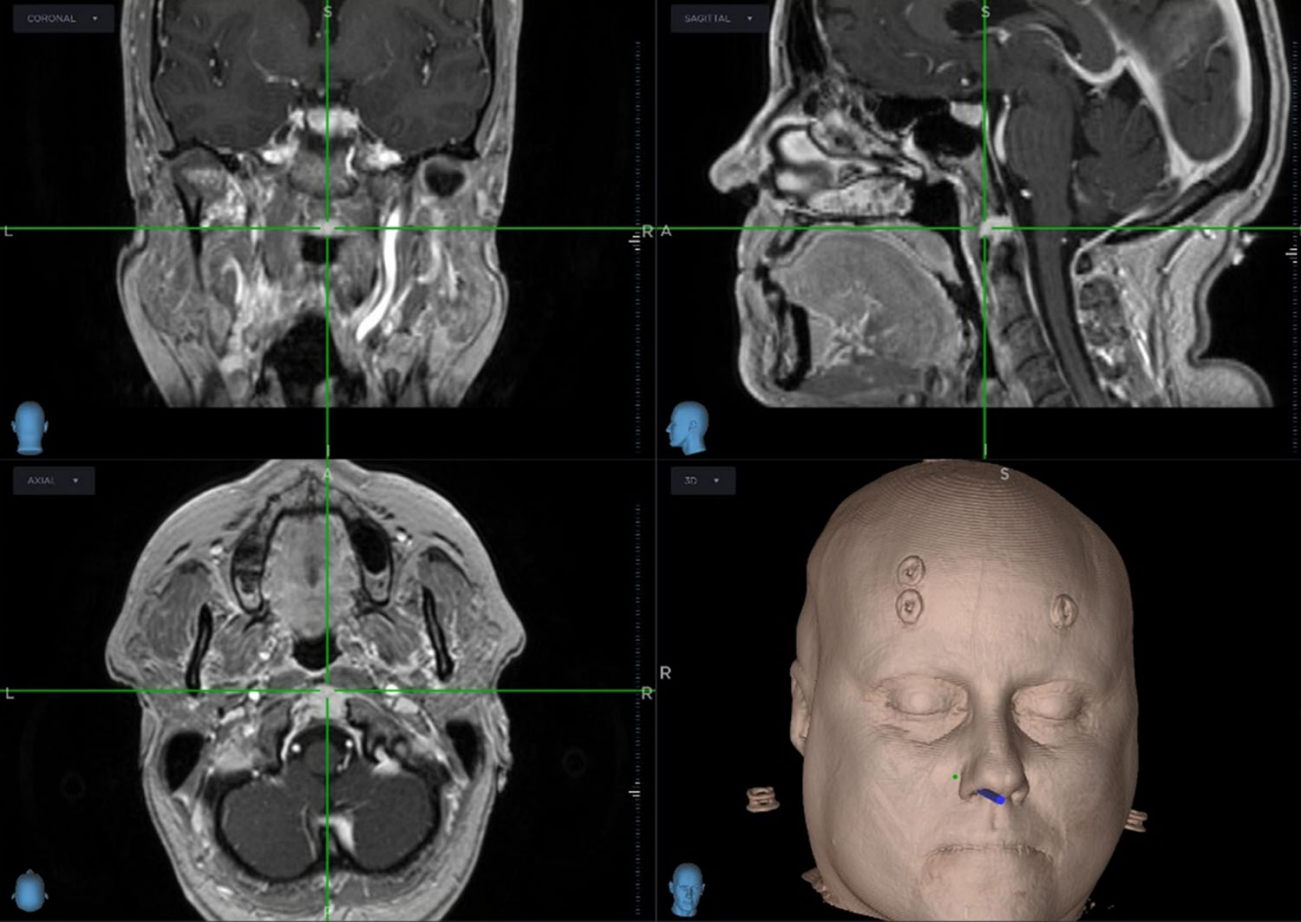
Case 1 stereotactic neuronavigational MRI. A soft tissue lesion was demonstrated and extended between the C1 and C2 articulation to the clivus. This was bound anteriorly by the anterior longitudinal ligament and posteriorly by the tectorial membrane. This measured 1.8 × 1.3 × 0.8 cm. The lesion was T2 hypointense and T1 isointense to muscle and did not demonstrate any diffusion restriction.
